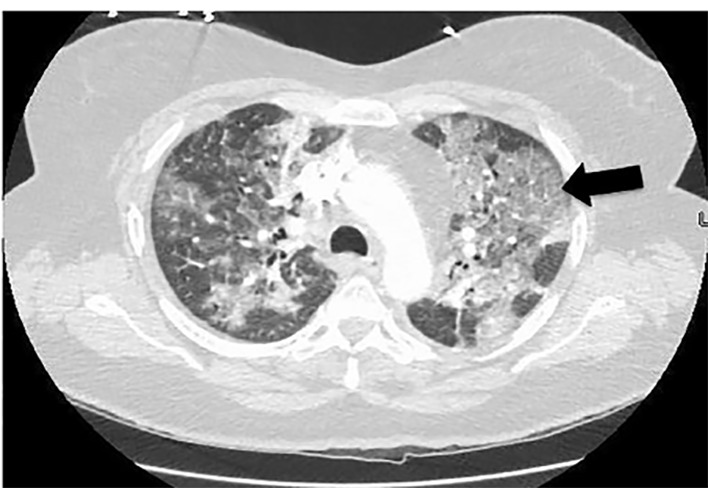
Crazy-paving pattern noted on computed tomography chest of coronavirus disease 2019 patient as manifested by multiple, patchy ground-glass opacities with reticular and interlobular septal thickening and intralobular lines in the axial plane. Crazy-paving pattern can be seen in both lung fields, but the tile-like or stone pavement resemblance pattern is best noted in the left lung (arrow).
